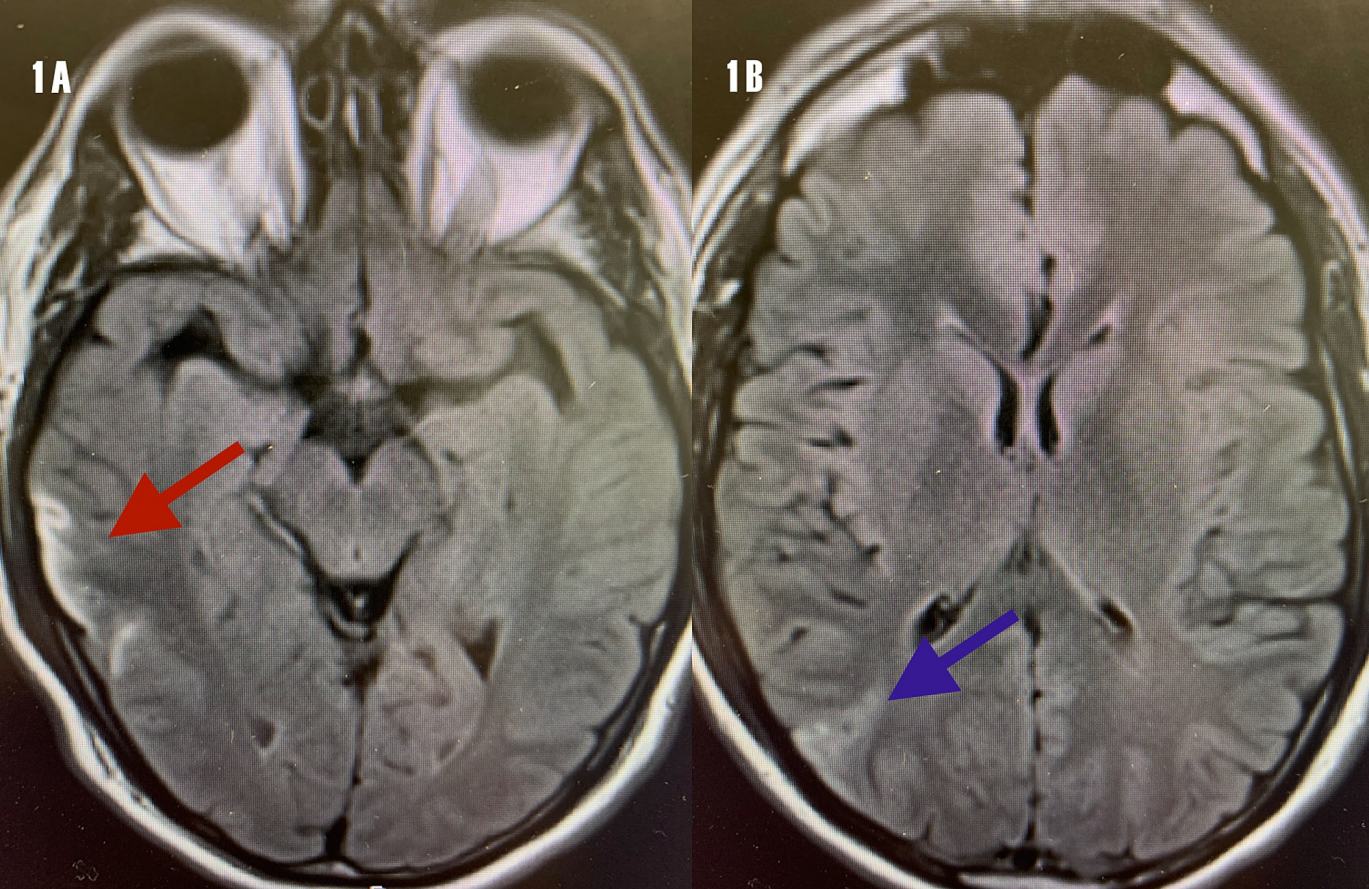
FLAIR MRI. (A) Right cortical temporal ischemic infarct (red arrow). (B) Right posterior parietal cortical ischemic infarct (blue arrow). FLAIR MRI, fluid-attenuated inversion recovery magnetic resonance imaging
